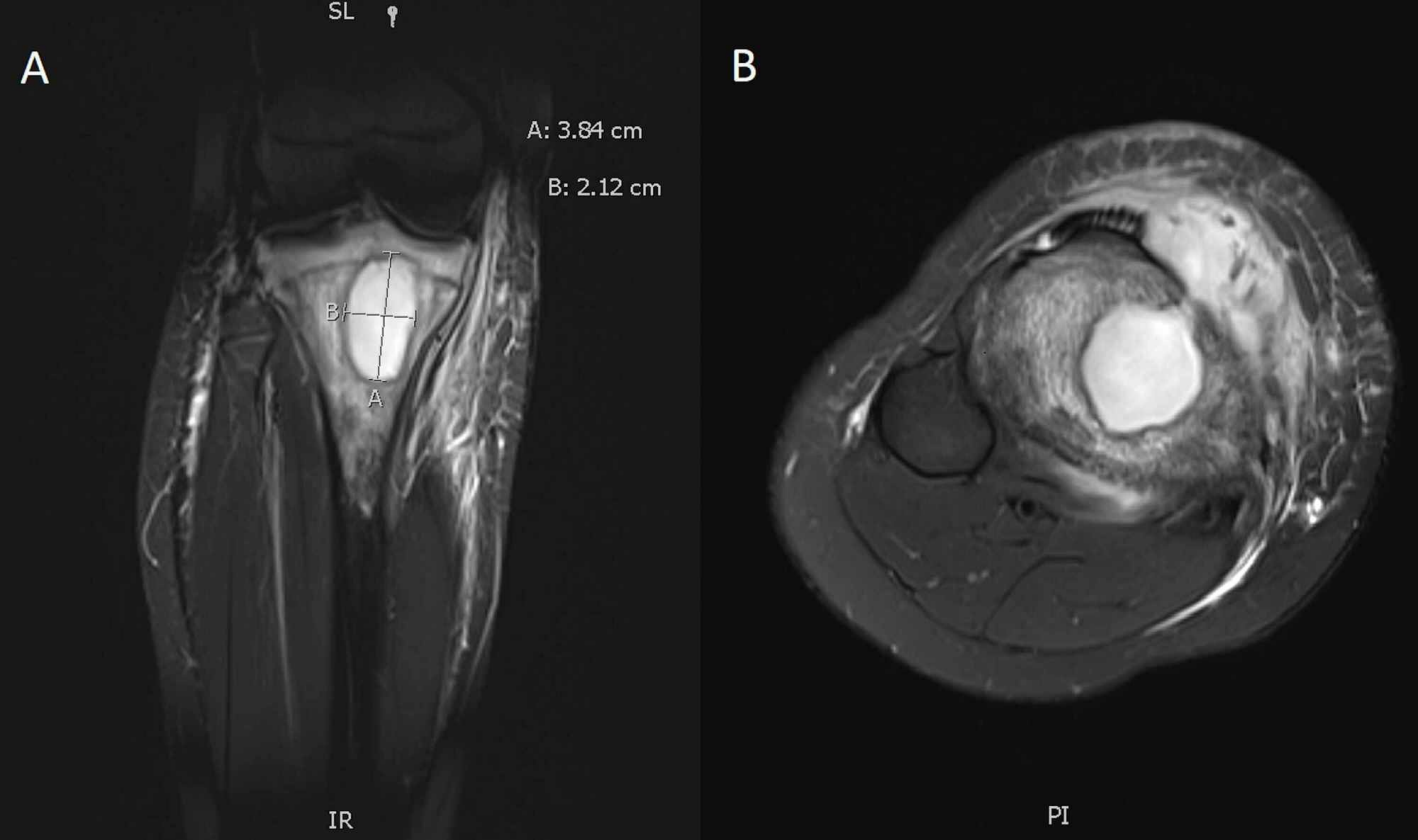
(A) Coronal view of T1 MRI with contrast demonstrating Brodie’s abscess in the right proximal femur. (B) Axial view of T1 MRI with contrast demonstrating Brodie’s abscess in the right proximal femur with a break in the cortex and extravasation into the surrounding tissue.
